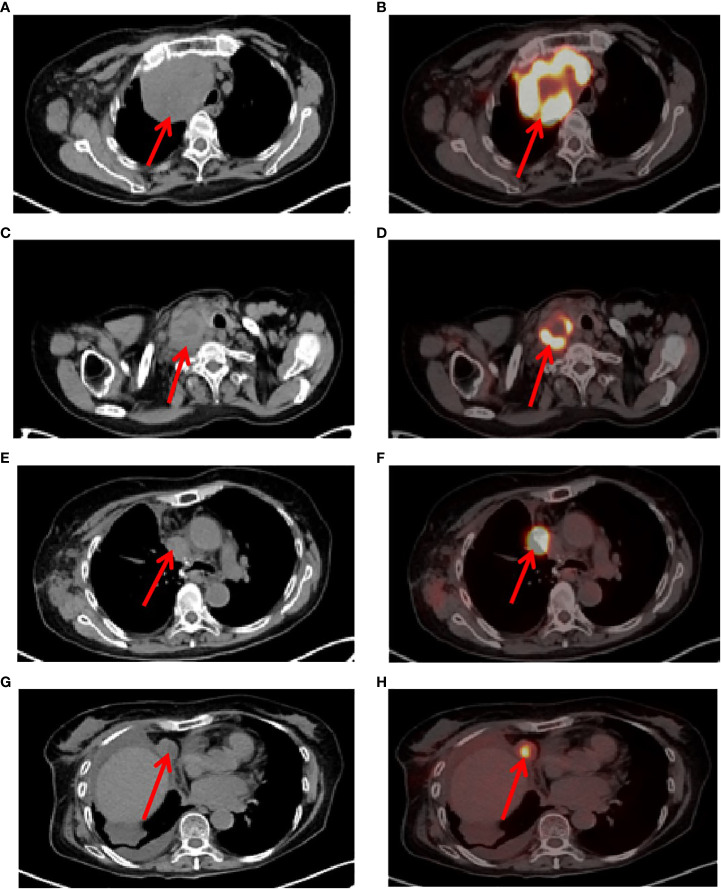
Tumor recurrence and metastasis region. (A, B), 7 weeks after surgery, positron emission tomography/computed tomography (PET-CT) revealed tumor recurrence and metastasis, involving a huge mass shadow in the right-side area of the mediastinum (solid tumor, dmax = 70 mm * 44 mm), abnormal increase in FDG metabolism, SUVmax 28, (C, D), Supraclavicular fossa lymph nodes, (E, F), right mediastinum lymph nodes, (G, H), right cardio-diaphragmatic angle metastatic lymph nodes.
